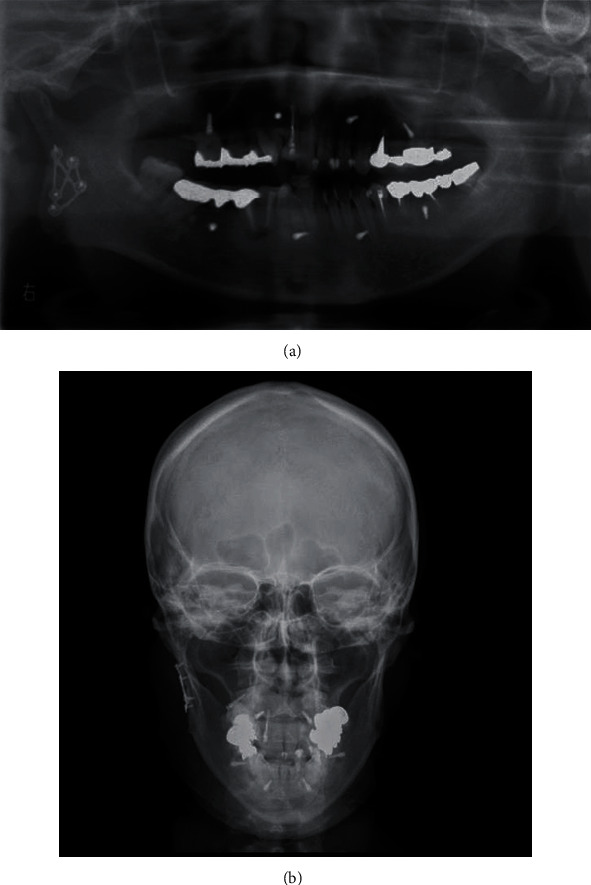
(a) Panoramic X-ray on the day after the surgery. The right mandibular condylar fracture is reduced and fixed. (b) Head X-ray (PA) on the day after surgery. The bone fragment that was displaced externally is reduced and fixed.
